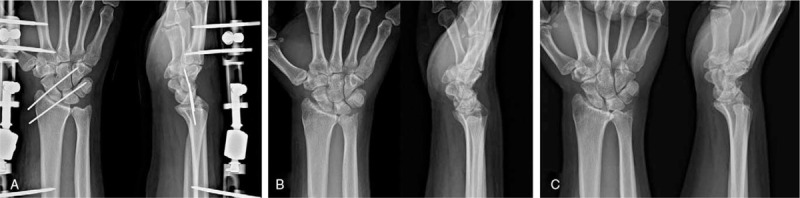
Postoperative plain radiographs. (A) Immediate postoperative (CUDR = 0.24), (B) 4 months postoperative (CUDR = 0.16), and (C) last follow-up at 22 months (CUDR = 0.18). CUDR = Carpal-ulnar distance ratio.
